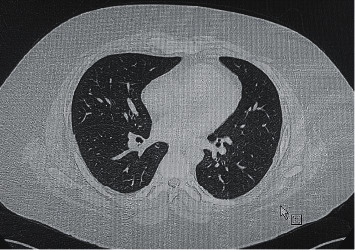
Posttreatment high resolution computed tomography.
